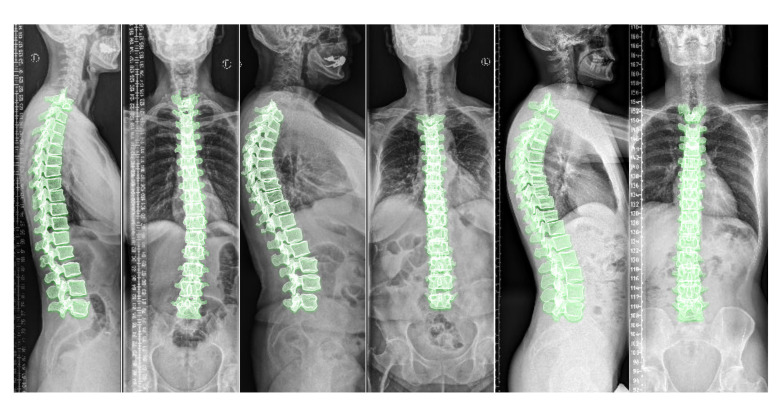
Full 3D spine models: 3D patient-specific spine models constructed from real clinical radiographs. Each sagittal and coronal view radiograph pair is from a different patient.
